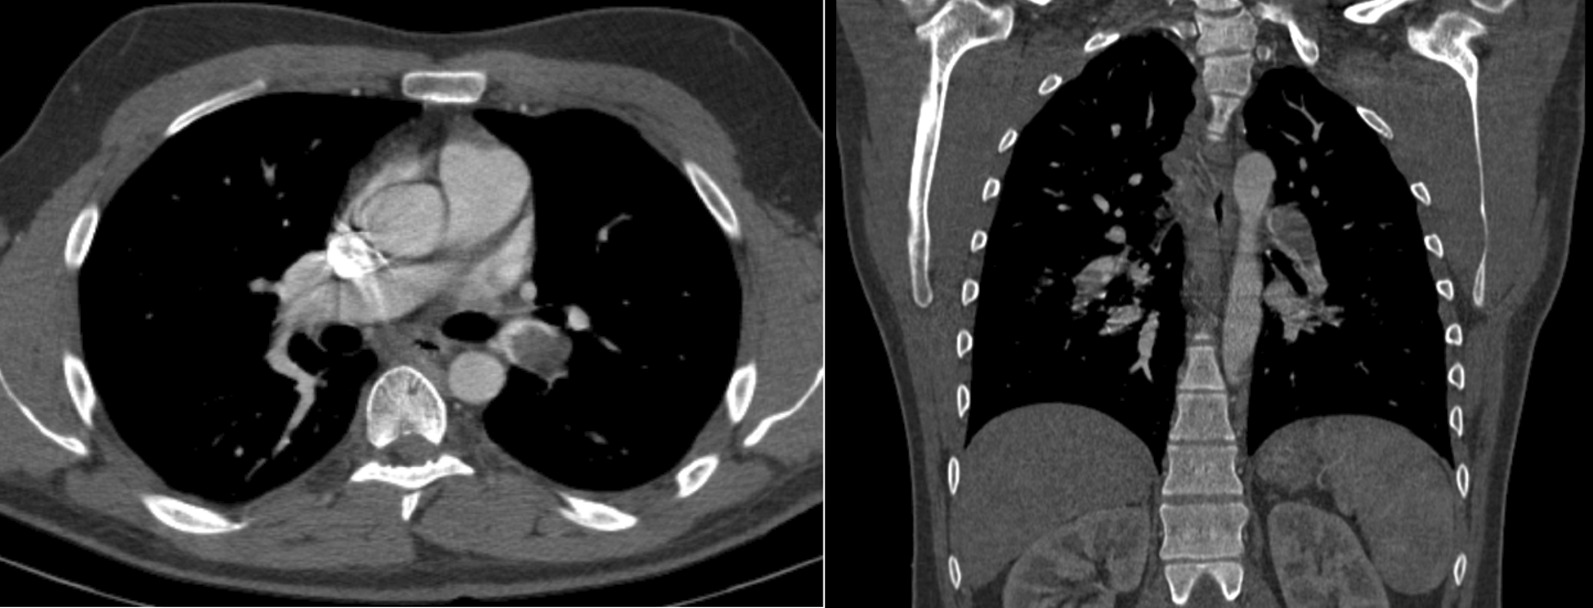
(Left) an axial slice from a CT of the chest obtained during the pulmonary angiographic phase of IV contrast administration demonstrates the largest site of PE in the patient, located in the left proximal interlobar pulmonary artery. (Right) a coronal view demonstrating PE located in the left proximal interlobar pulmonary artery
